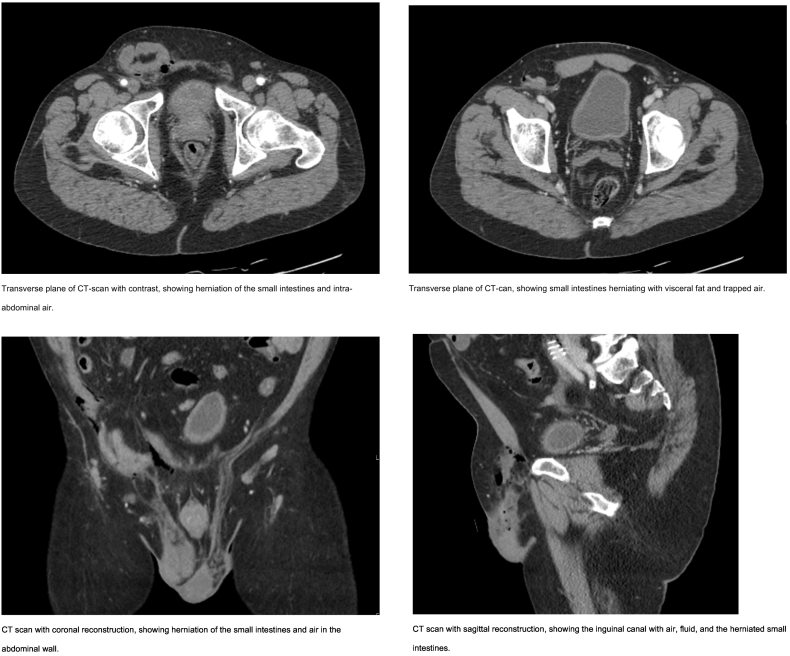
Patients CT images. Transverse plane of CT-scan with contrast, showing herniation of the small intestines and intra abdominal air. Transverse plane of CT-scan with contrast, showing herniation of the small intestines with visceral fat and trapped air. CT scan with coronal reconstruction, showing herniation of the small intestines and air in the abdominal wall. CT scan with sagittal reconstruction, showing the inguinal canal with air, fluid, and the herniated small intestine.
